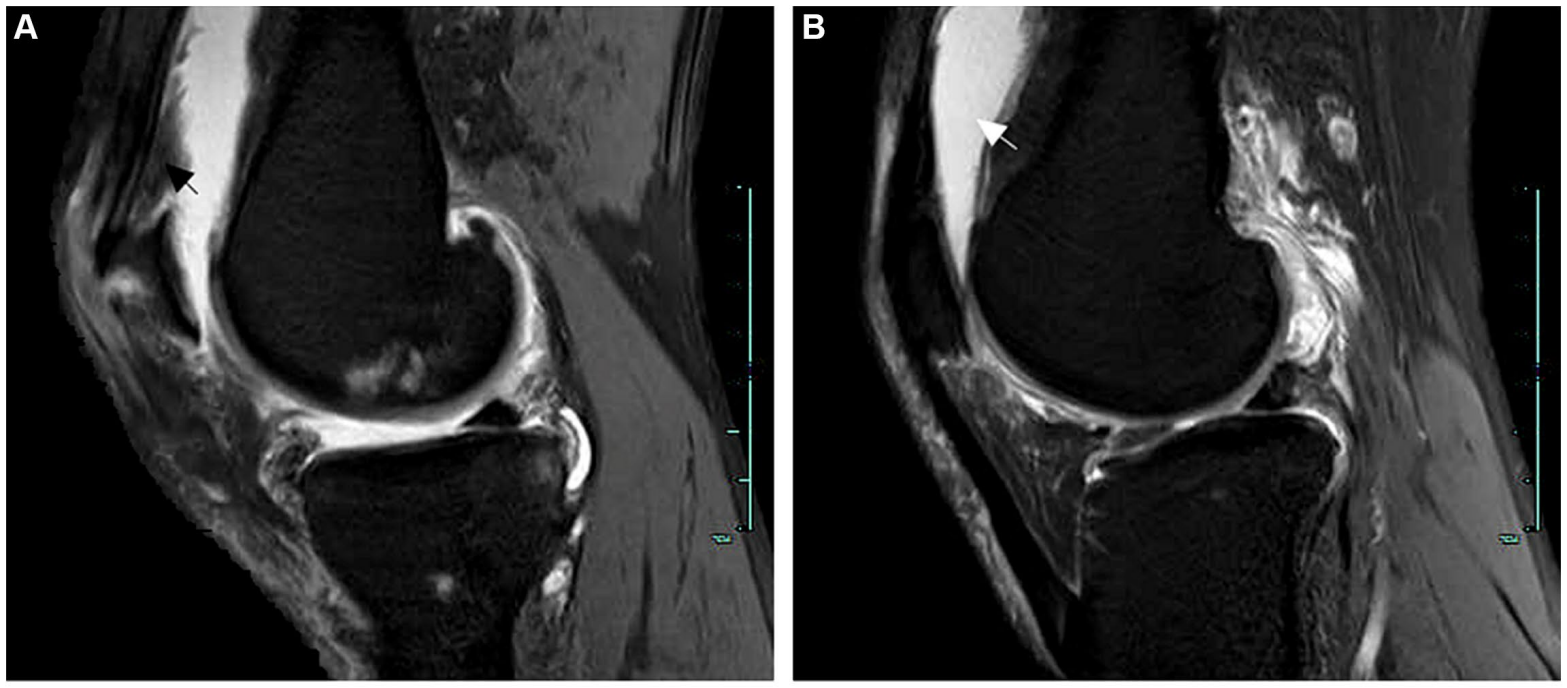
MRI examination of the right knee joint. (A) The arrow indicates synovitis. (B) The arrow points towards joint cavity effusion.
